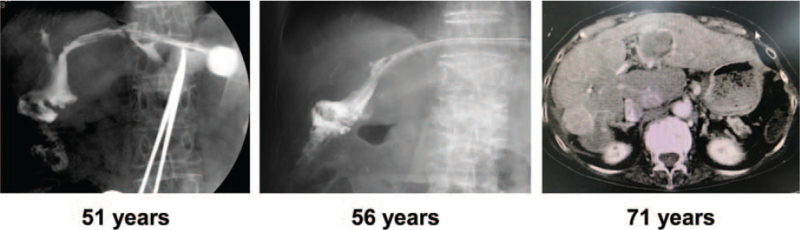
No bile ducts were initially visible on cholangiography. In a contrast-enhanced CT scan, multiple deposits of hepatocellular carcinoma in both lobes of the liver were visible.
